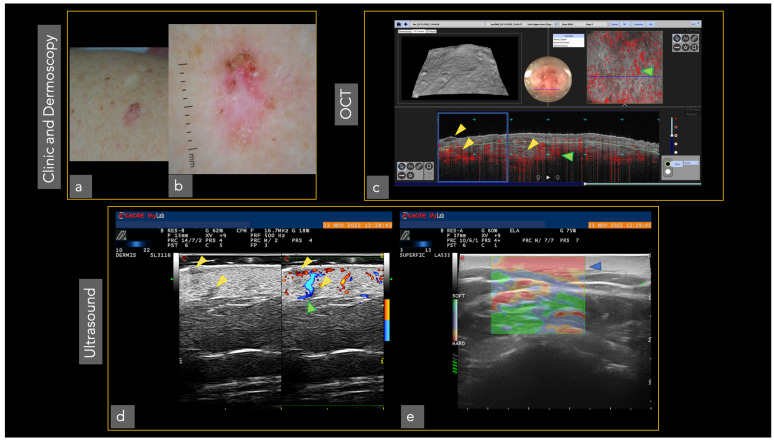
Superficial and infiltrating basal cell carcinoma in the left arm was studied with different techniques. Tumor presence (yellow triangles). The vascular pattern is represented with green triangles: (a) Clinical image; (b) Dermoscopy; (c) OCT showing superficial and infiltrating basal cell carcinoma (blue square). The tumor surface is perceived (superior left). Dermoscopy area scanned (center). The vascular pattern can also be observed in 3 D (superior right); (d) The same tumoral islands are observed in ultrasound (left). The addition of the Doppler image reveals the complete tumor invasion (right); (e) The elastography shows the denser area corresponding to the tumor (blue triangle). Ultrasound image courtesy of Dr. Priscila Giavedoni.
